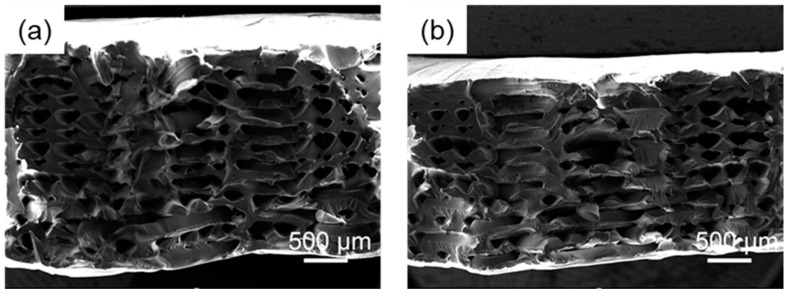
Tensile section, (a) 0.25 s second ultrasonic sample, (b) 0.5 s first ultrasonic sample.
